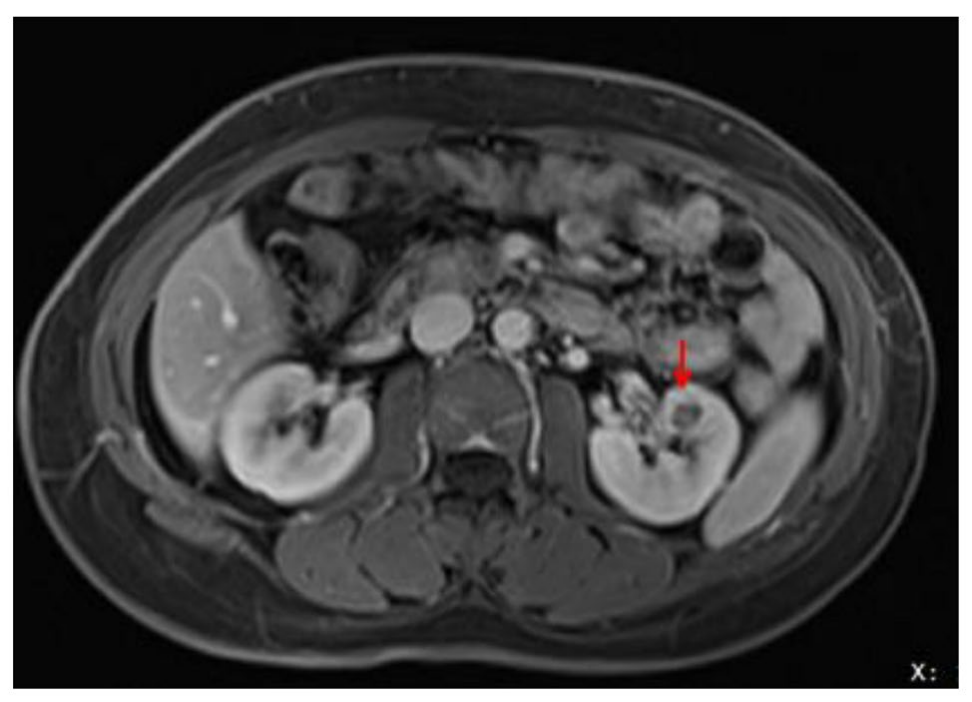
Magnetic resonance imaging (MRI) shows a mass in the left kidney (red arrow)
